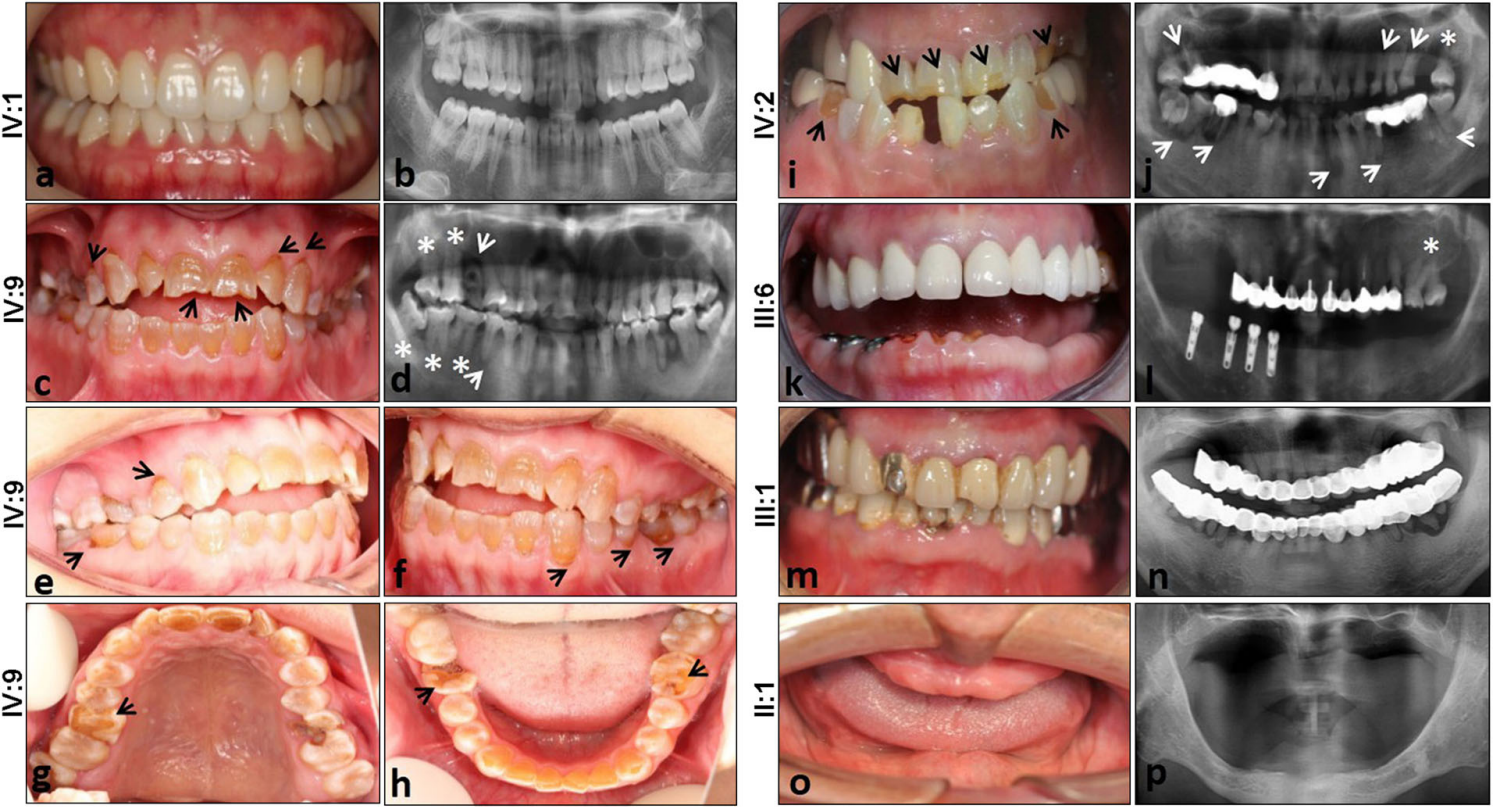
Dental phenotype and panoramic radiograph of the patients. a Normal permanent teeth; b panoramic radiograph of normal teeth. Dental phenotype of IV:9 c,e–h. An intraoral view of a male patient at the age of 21 shows brown crowns with thin and chipping enamel, especially in the cervical part of all teeth (black arrows), and short crowns of the permanent maxillary central incisors worn out by attrition (black arrow). d,j,l Panoramic radiographs of patients show bulbous crowns covered with thin enamel, cervical constriction (asterisk), short constricted roots, and apical radiolucencies in the second molars (white arrows). i An intraoral view of a patient at the age of 22 shows grey crowns with enamel chipped and absent in places. k,m Above the age of approximately 40, patients had lost almost all of their teeth or had several loose roots. l,n Patients were treated by long-span fixed partial denture repair, l implant prosthodontics, k removable partial denture, or o removable complete denture according to their family economic situation. Finally, o,p all patients lost all their teeth at an early age (generally by their 50s).
